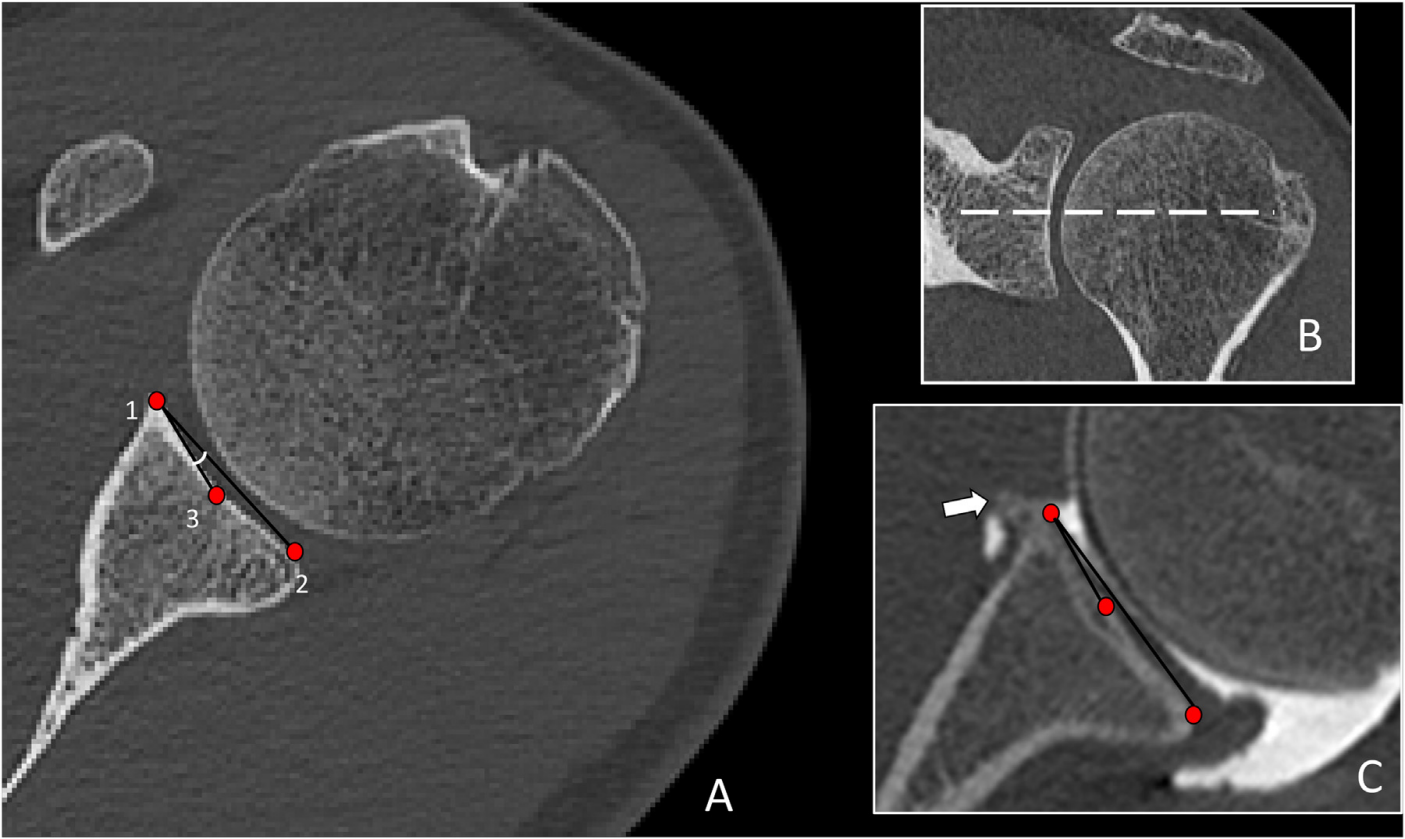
A) Glenoid concavity angle (GCA) measurement on an axial CT image passing through the center of the glenoid of a 33 year-old male with no clinical signs of shoulder hyperlaxity. The angle formed by the lines connecting points 1–2 and that connecting points 1–3 corresponds to the GCA. B) The dotted line on the upper left image shows the corresponding level in the coronal plane. In case of bone changes such as osteophytes, these are not taken in consideration when measuring the GCA. C) Correct measurement of the GCA on a 39 year-old patient with hyperlaxity, giving a GCA of 2° Osteophyte is visible at the anterior articular rim (arrow) and is not used for measurements.
